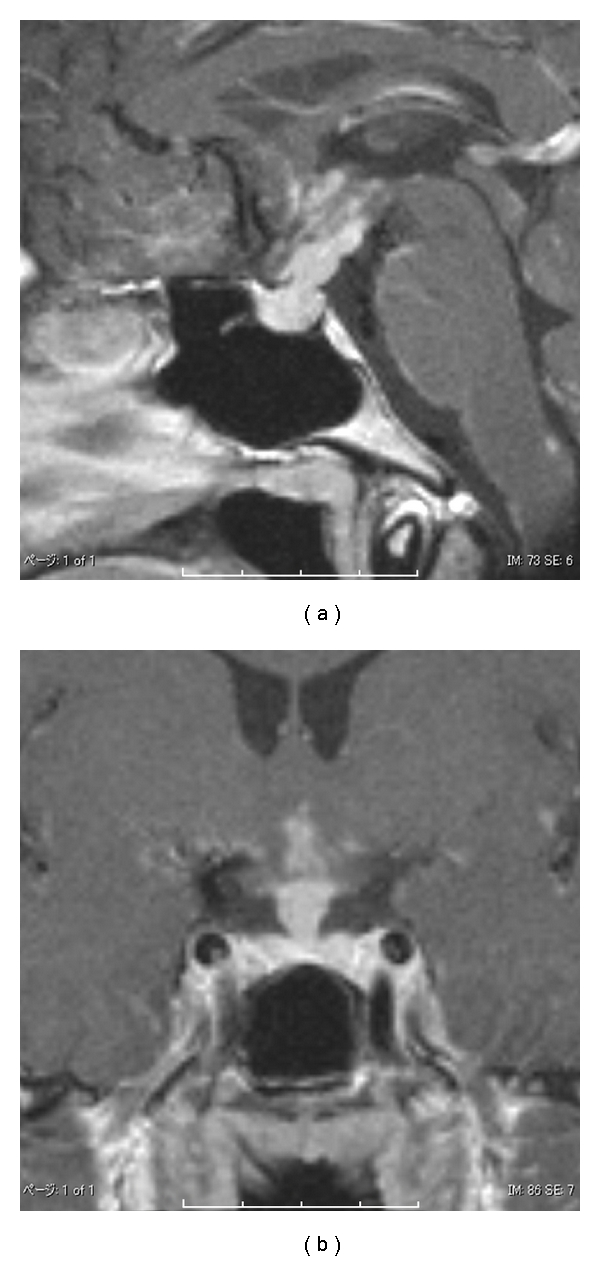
T1-weighted MRI of the sella turnica after administration of gadolinium revealed enhancing lesion centrally in the pituitary stalk, which spread continuously to the bottom of the third ventricle, and high intensity of posterior lobe was lost.
